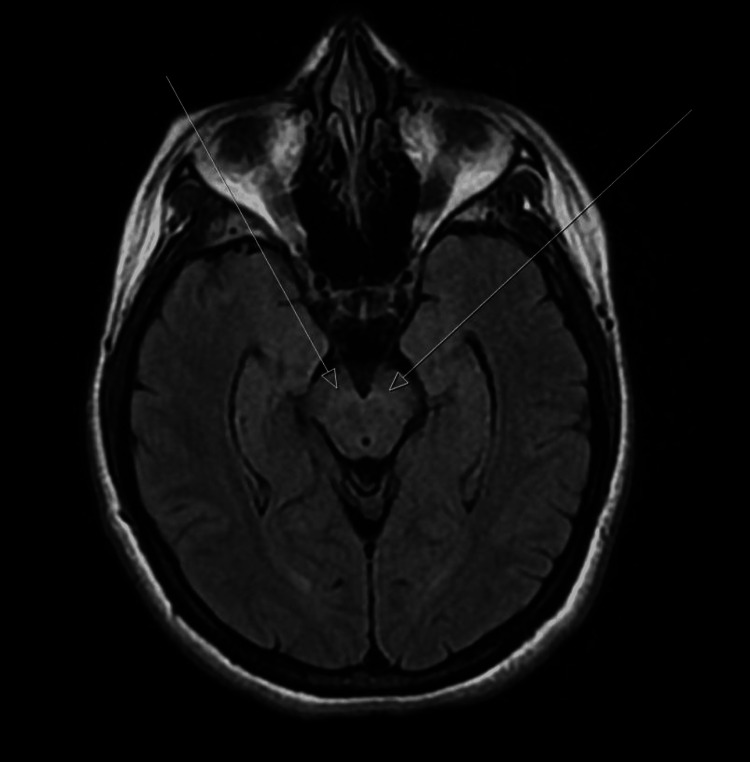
MRI brain showing symmetric T2 hyperintense signal abnormality within the midbrain between the red nuclei and cerebral peduncles (arrows), which is a sign of potential demyelinating disease.
